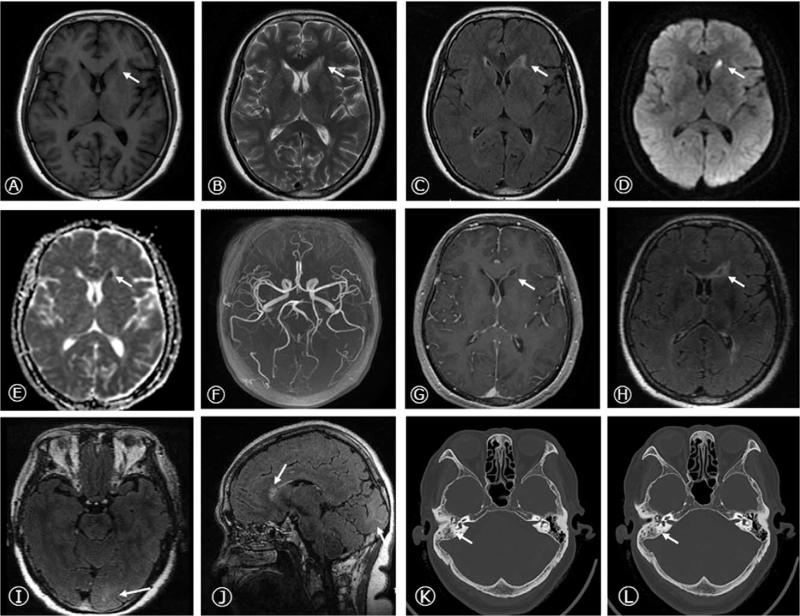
Brain MRI and Temporal bone CT at admission. Axial T1-weighted sequence, T2 -weighted sequence, FLAIR sequence (A-C), DWI (D), and ADC (E) sequences were imaged and showed abnormal signals in the left head of caudate nucleus, next to the anterior horn of lateral ventricle. No significant abnormality was observed in the cerebral MRA (F). Cranial SPGR and meningeal CUBE enhancement displayed the ring-enhancement in the left head of caudate nucleus and meningeal linear enhancement in left occipital lobe on axial (G-I) and sagittal images (J). The temporal bone CT showed the right otitis mastoidea (K, L). CT = computed tomography, MRI = magnetic resonance imaging.
